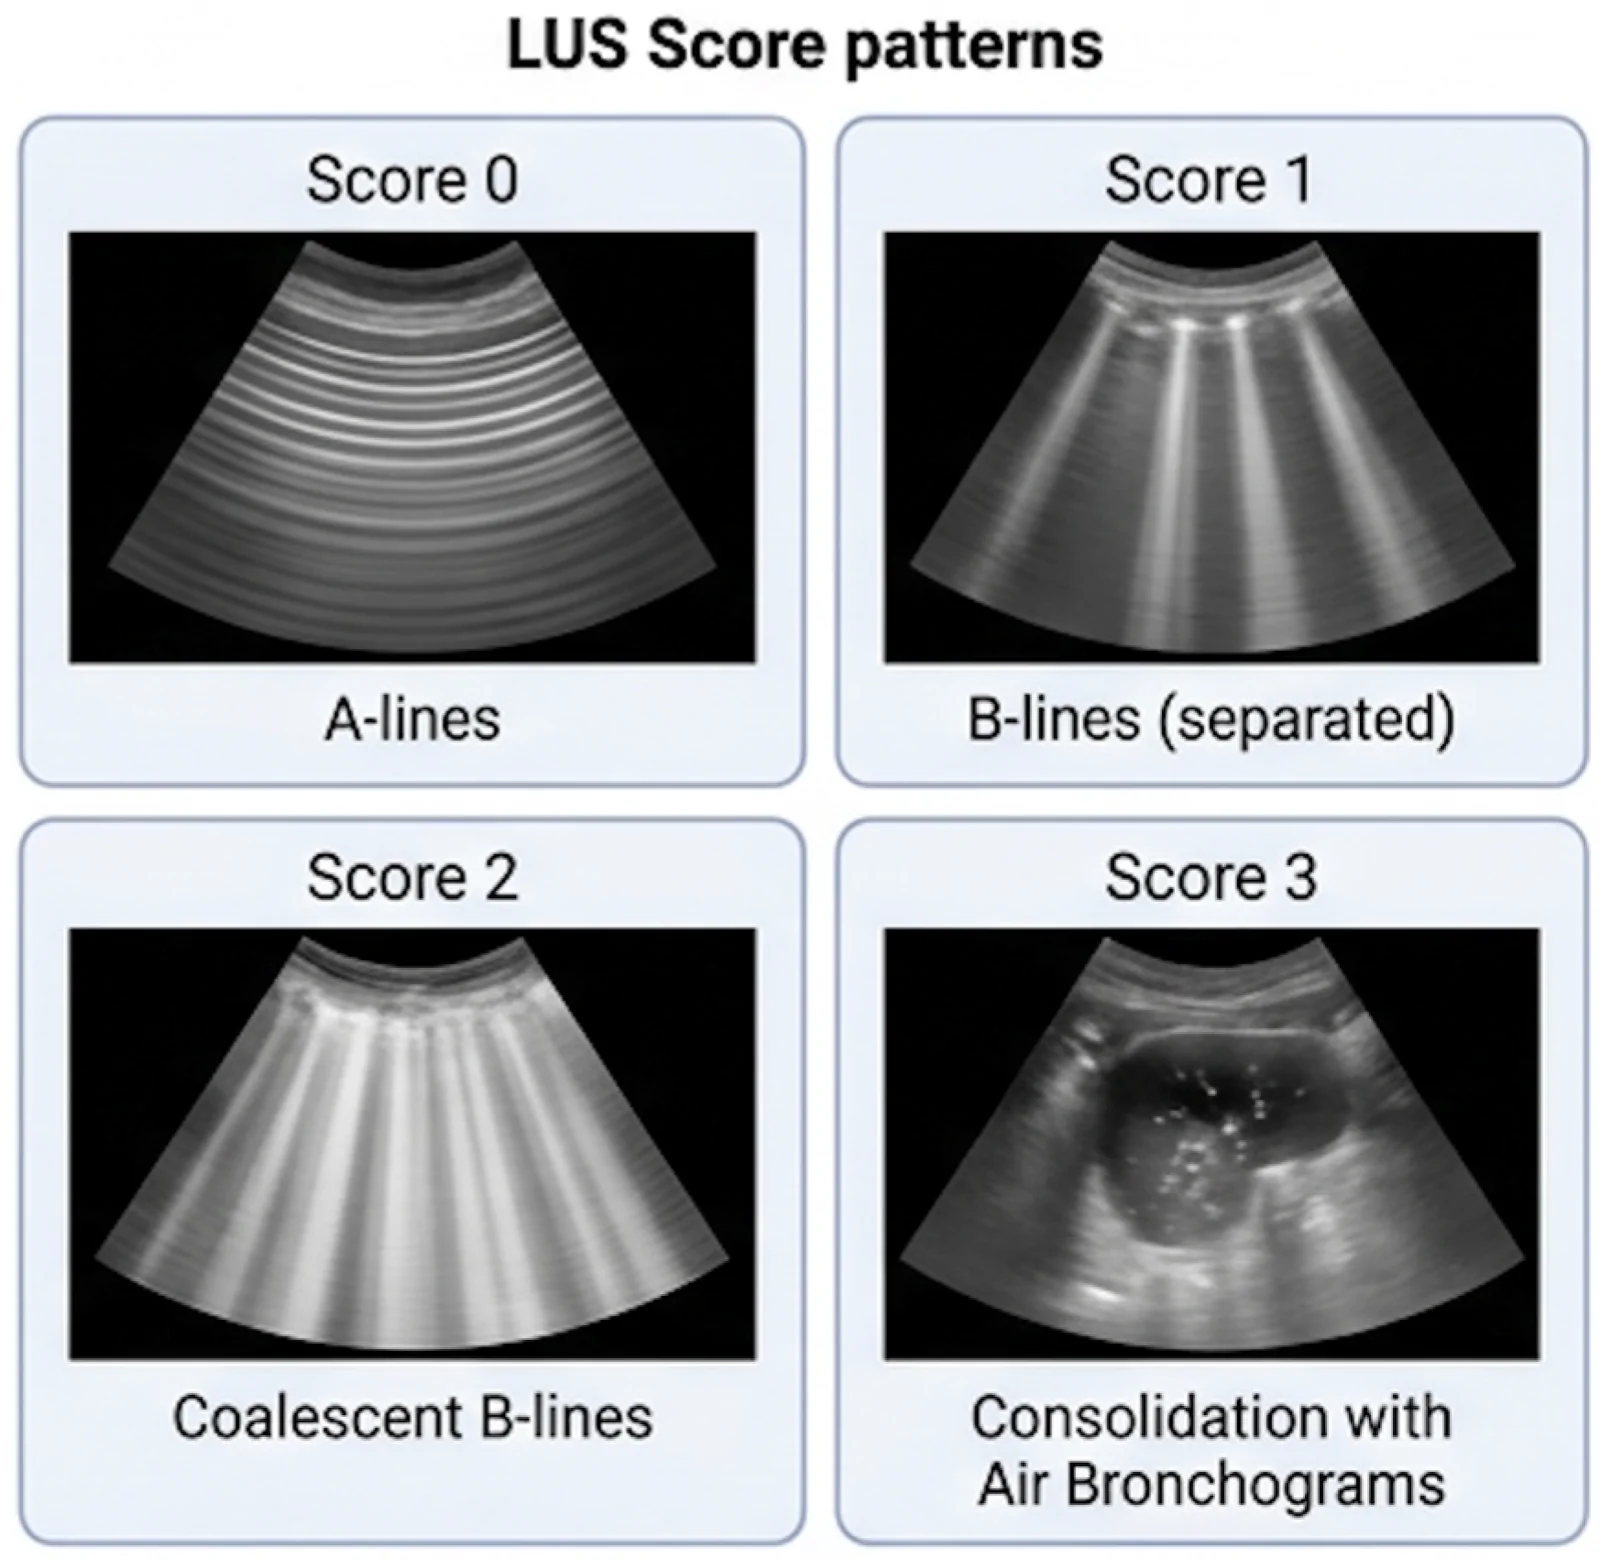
Representative lung ultrasound patterns corresponding to the Lung Ultrasound Score. Representative sonographic appearance of the four semi-quantitative LUS score categories (scores 0–3) to assess peripheral lung aeration in preterm infants. Figure created using FigureLabs https://www.figurelabs.ai/ accessed on 7 July 2026.
